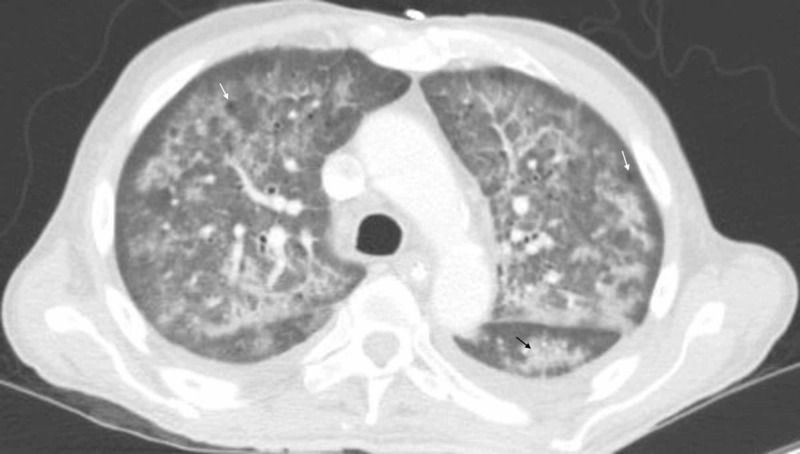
Contrast-enhanced CT of chest. Bilateral alveolar opacities ( black arrow ), pneumatoceles ( white arrows ), and bilateral small pleural effusions.
